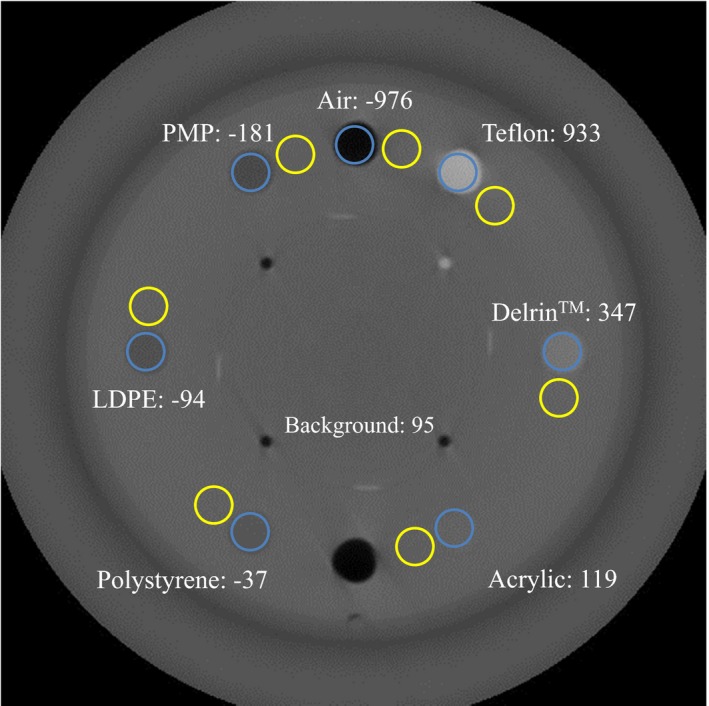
DCBCT image of the HU reproducibility module (CTP 404) with seven inserts of materials with different densities. The blue circles are the ROIs inside the inserts and the yellow circles are the ROIs surrounding the inserts and are used to measure background. Numbers are the HU values from diagnostic CT.
